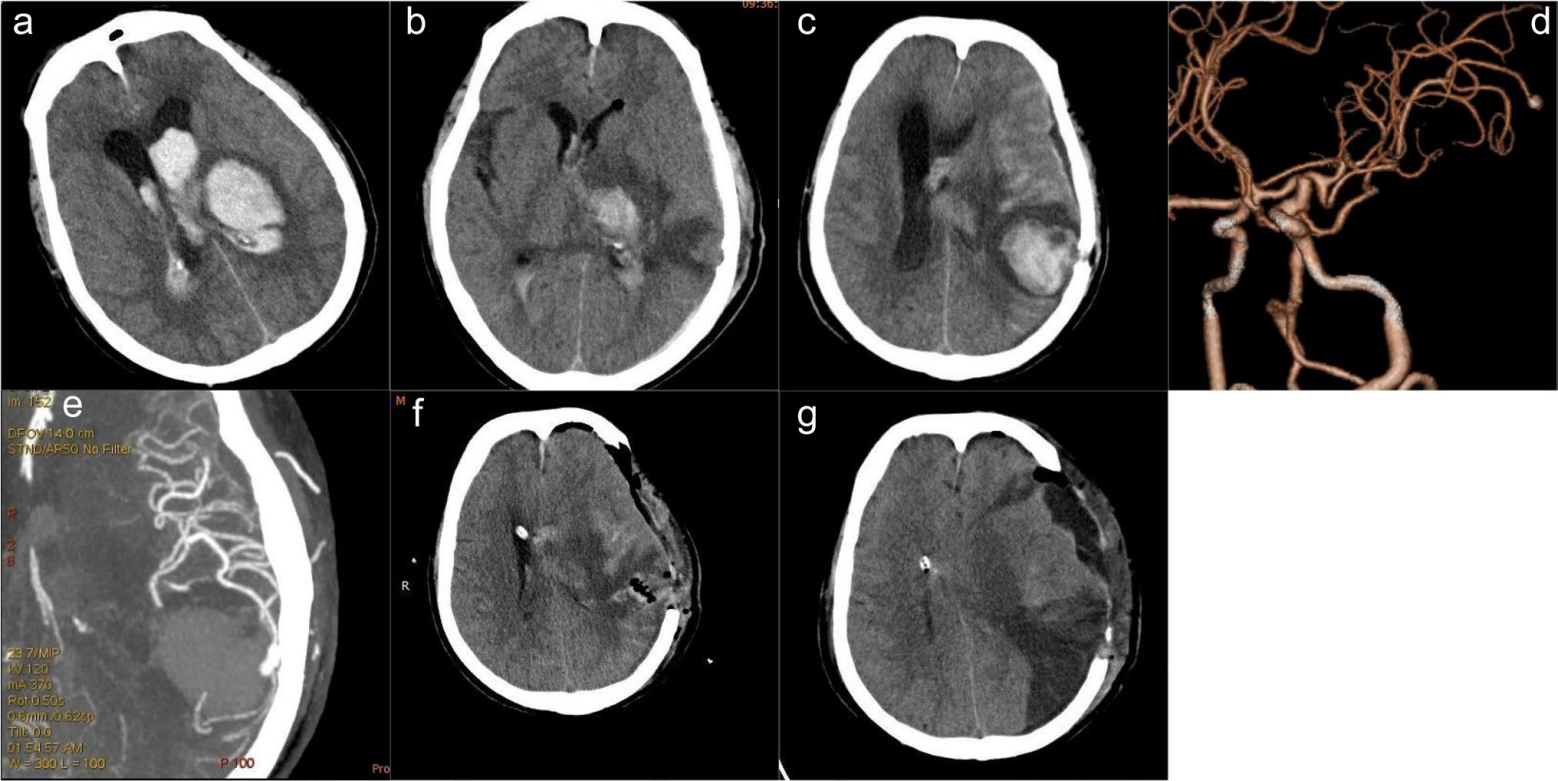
Neuroimaging acquired from our patient: a axial head computed tomography on initial patient presentation showing left thalamus hemorrhage, intraventricular extension, and hydrocephalus; b CT scan performed on the 6th postoperative day showing that the TH was almost eliminated; c CT scan performed on the 18th postoperative day showing a new intraparenchymal hemorrhage with 6mm of midline shift; (d, e) computed tomography angiography(CTA) showing a left distal middle cerebral artery pseudoaneurysm (red arrow); f CT scan performed on 8-h post-craniotomy showing that the hemorrhage had been evacuated completely and the bilateral ventricles had shrunk; g CT scan performed on the fourth day after the post-craniotomy showing left parietal lobe cerebral infarction
